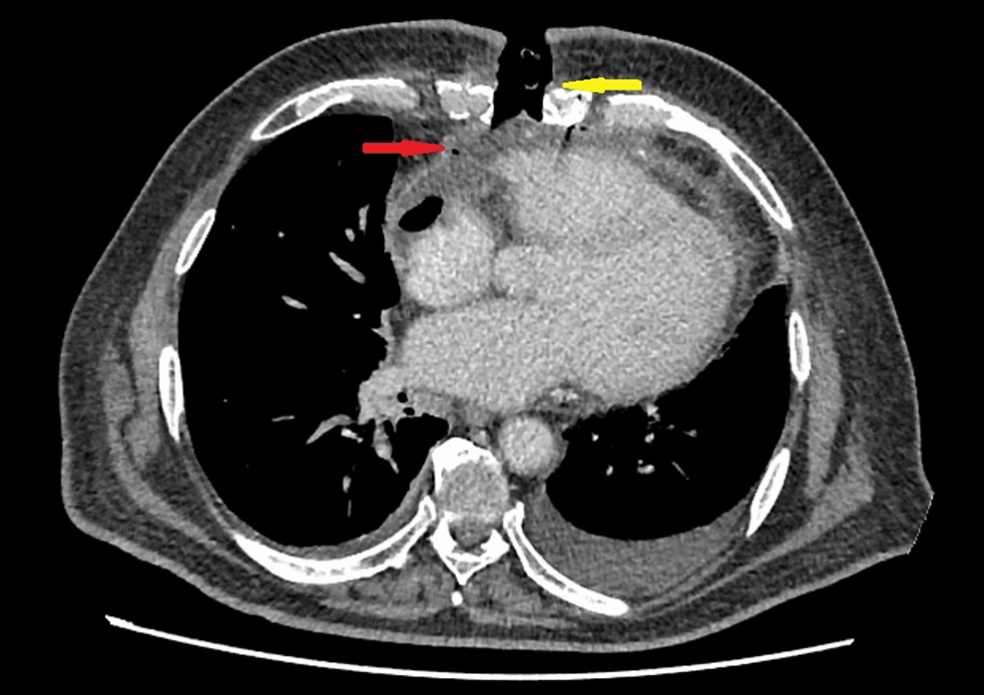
CT scan (axial cut) showing the midline sternotomy wound defect and sternal dehiscence (yellow arrow), with features of mediastinitis evidenced by retrosternal fluid and free air locules (red arrow).
